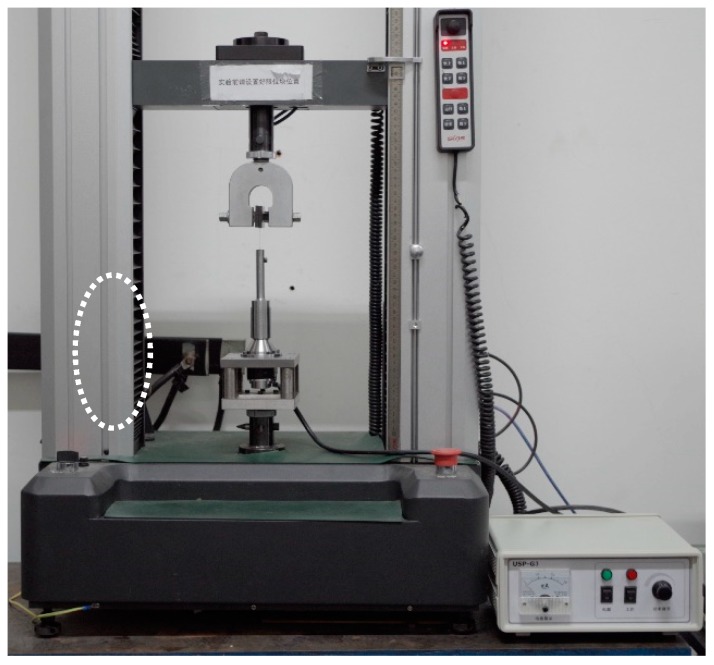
Ultrasonic-vibration-assisted uniaxial tension device.
